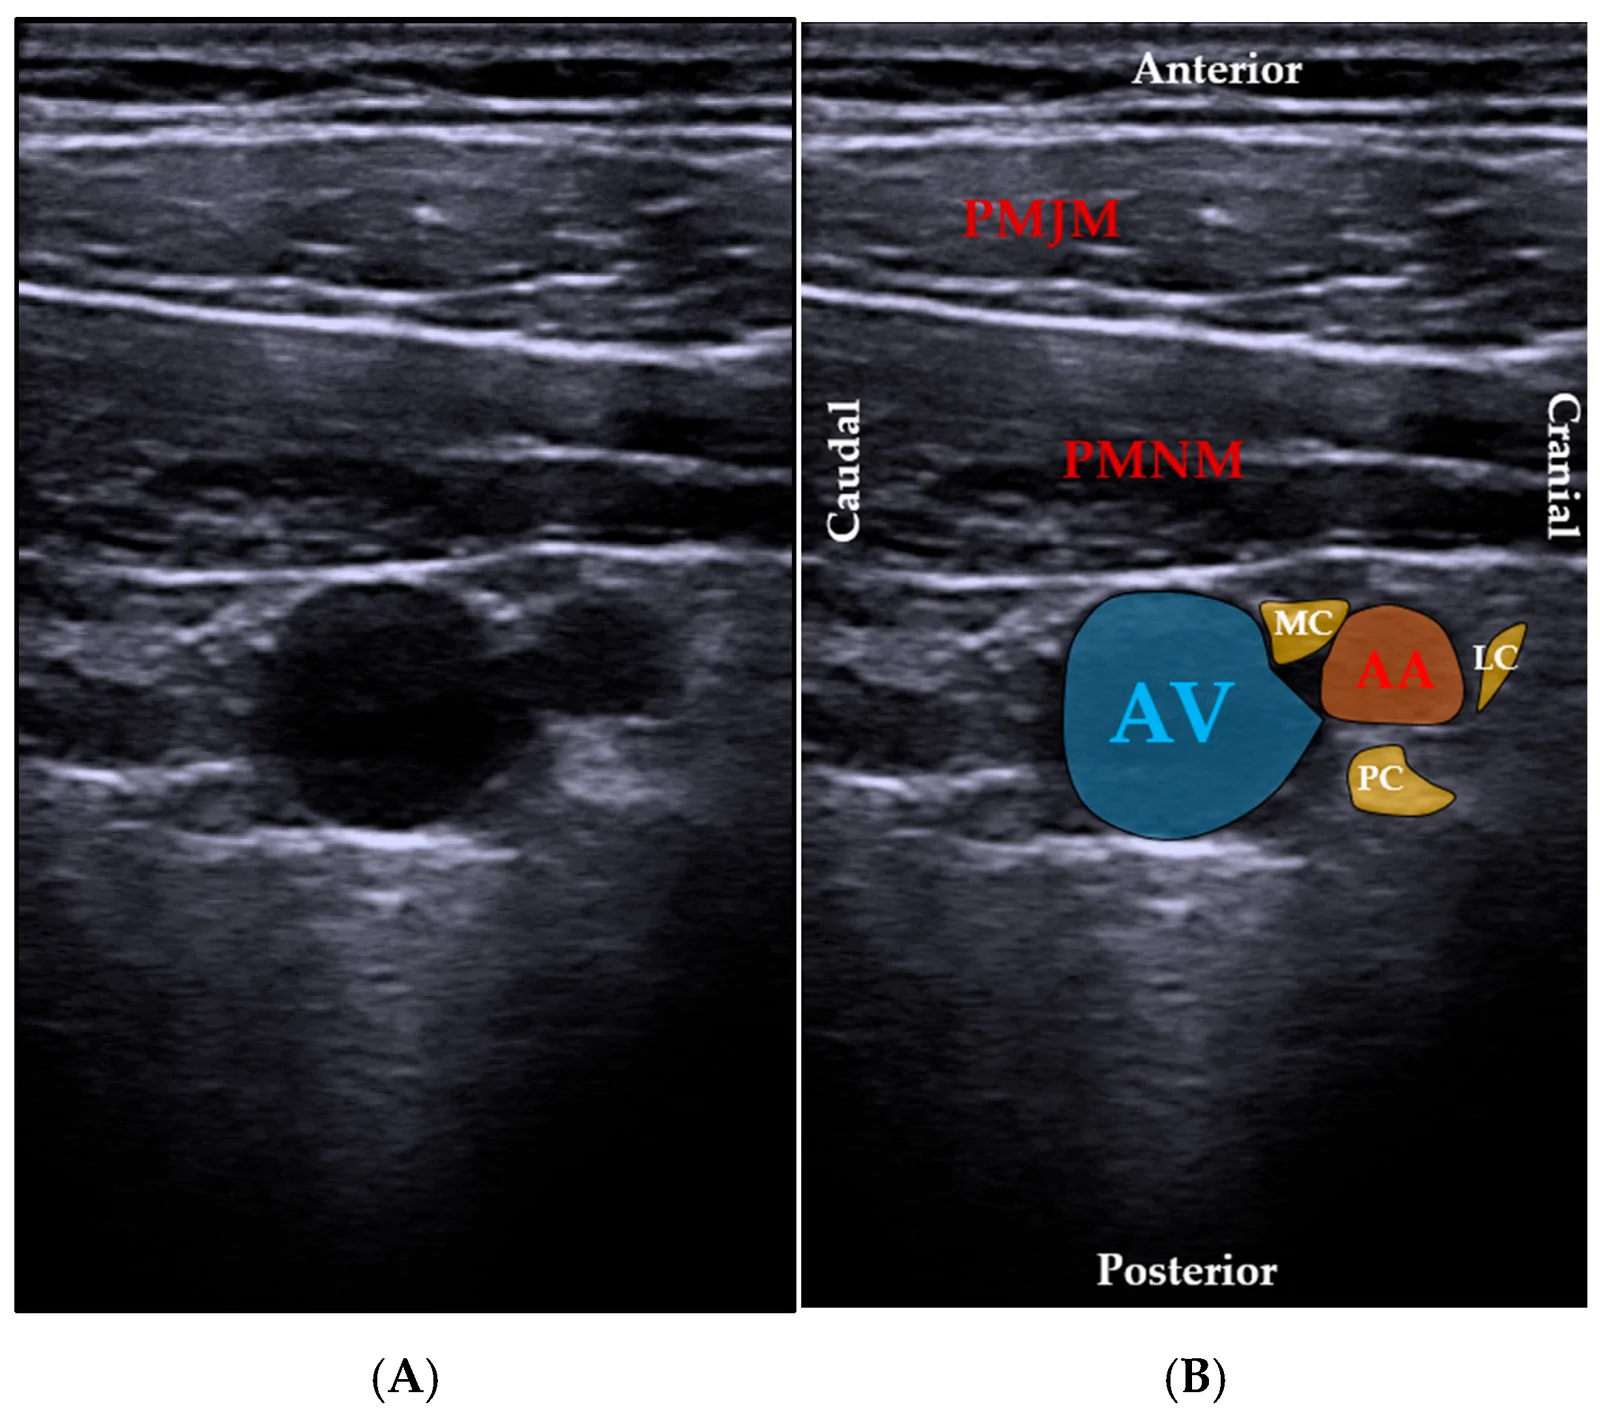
Ultrasound images of the brachial plexus cords, axillary artery and axillary vein, and the pectoralis major and pectoralis minor muscles in the infraclavicular region. (A). Unannotated ultrasound image of the infraclavicular region. (B). Ultrasound image of the infraclavicular region with the identified anatomical elements. Legend: PMJM—Pectoralis major muscle, PMNM—pectoralis minor muscle, AA—Axillary artery, AV—Axillary vein, LC—Lateral cord, MC—Medial Cord, PC—Posterior Cord.
